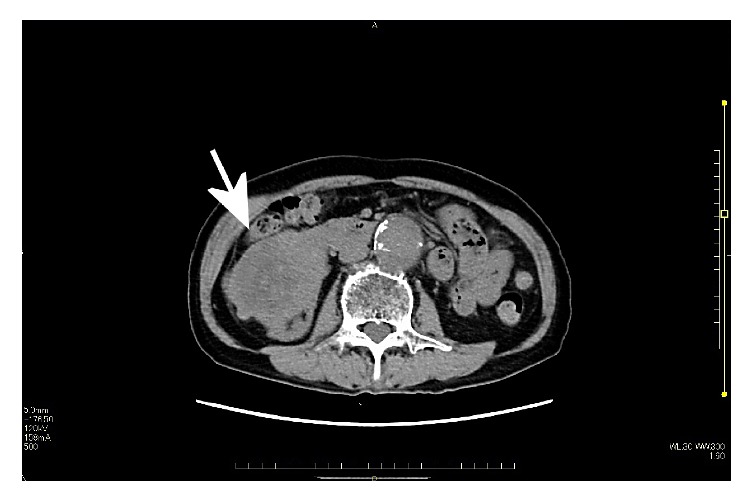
CT image showed right renal mass (arrow).
